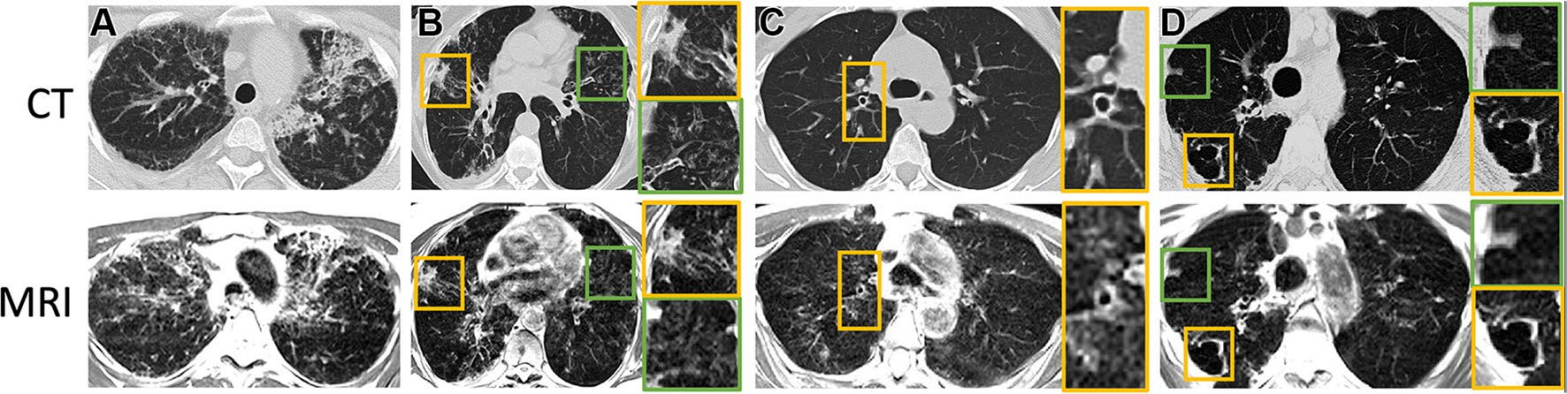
Axial CT (reformatted to 0.8 × 0.8 × 6 mm) and T2-weighted MR (reformatted to 1.1 × 1.1 × 6 mm) images show A honeycombing, interstitial thickening, and fibrotic changes in a 35-year-old woman; B consolidative opacities in a 70-year-old woman; C bronchial wall thickening with bronchiectasis in a 58-year-old woman; and D a cavitary lesion in a 70-year-old woman. B–D Green and yellow boxes on CT and MR images denote the area of interest around the particular finding described, with the corresponding box bordered in that color to the right of the respective image showing an enlarged view of the area. Image is reproduced from Campbell-Washburn et al. [57]
